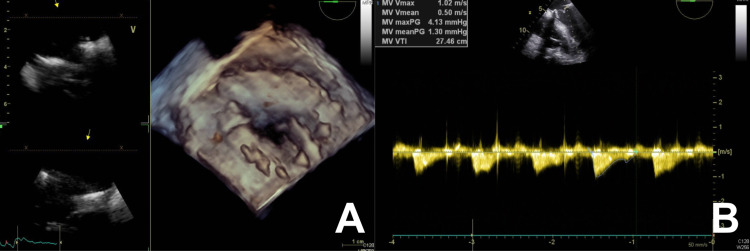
(A) 3D transesophageal echocardiogram (TEE) and (B) TEE with Doppler demonstrating annuloplasty sutures and recurrent mitral valve regurgitation.
